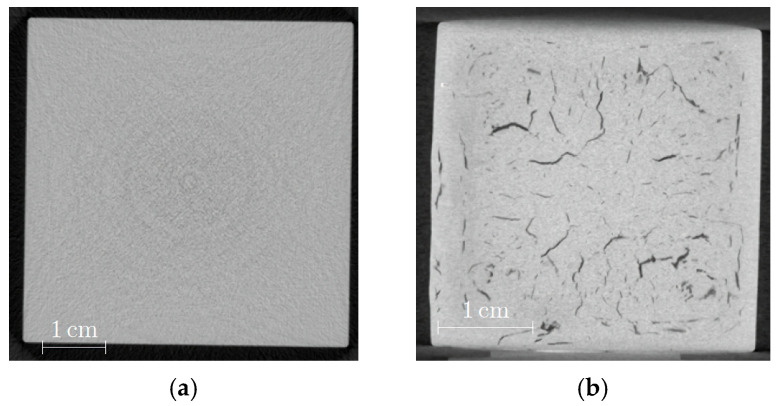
CT images of cuboid with a cross section in the middle: (a) green body; (b) sintered state.
